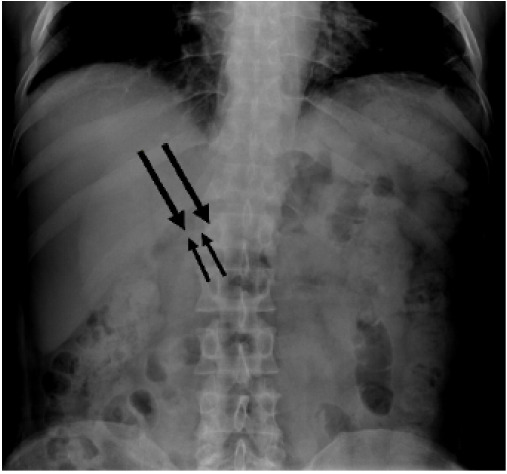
Anterior-posterior abdominal radiograph showing the foreign body (arrows).
